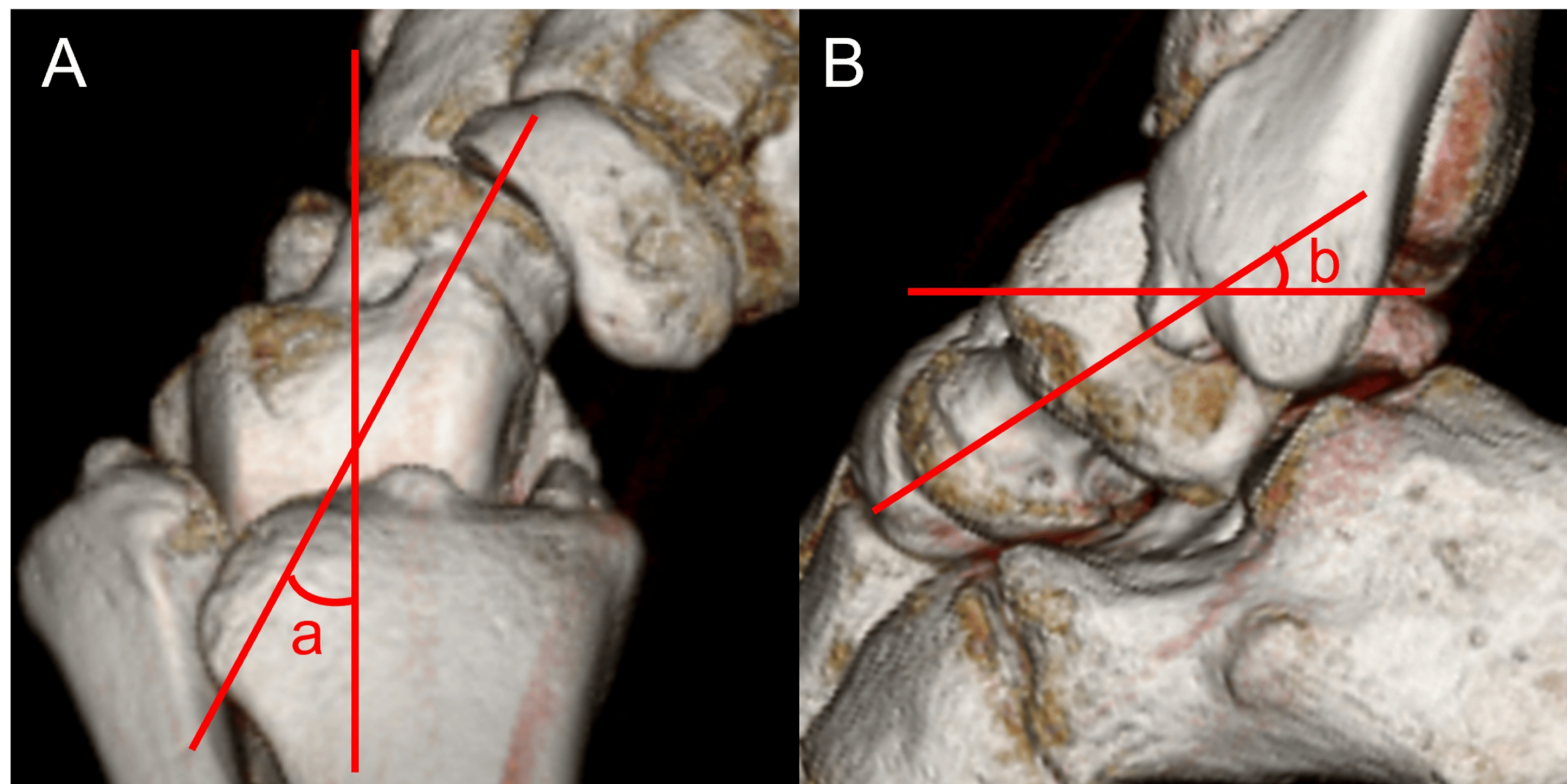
Three-dimensional computed tomography of the talus (A) Axial view, (B) sagittal view. (a) The declination angle of the talar neck relative to the body; (b) the inclination angle of the talar neck relative to the body
